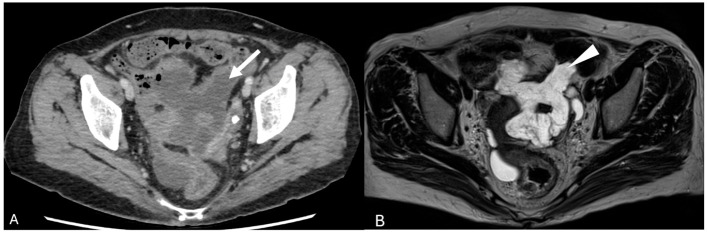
Axial contrast enhanced CT and T2 weighted non-fat saturated images of the pelvis. The locally invasive cecal cancer demonstrates low attenuation on CT (arrow, image (A)) which is a nonspecific finding but in this case corresponds to mucin. The correlate image acquired at the same level on MRI demonstrates the correlate of T2 hyperintense signal in >50% of the tumor consistent with a mucinous tumor (arrowhead, image (B)).
